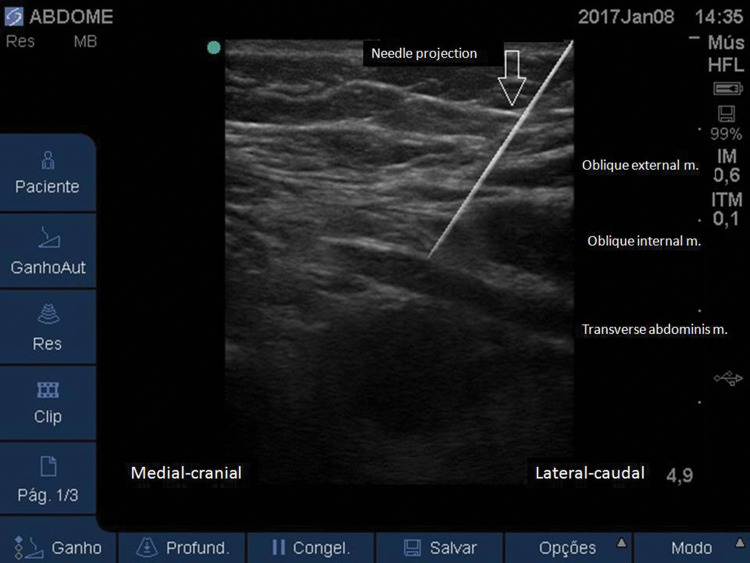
Ultrasound image showing the proper position of the needle tip in the plane between the IO and TA muscles.
